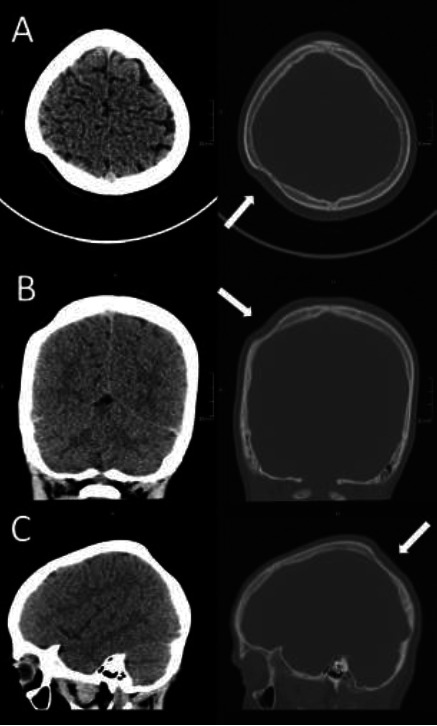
Non-contrast CT-scan in the 3 axes in the bone and tissue windows showing the thinning of the skull (arrows) and the normal appearance of the underlying brain tissue. Image A represents an axial view with tissue windowing (left image) and bone windowing (right image), image B represents a coronal view with tissue and bone windowing, and image C represents a sagittal view with tissue and bone windowing
